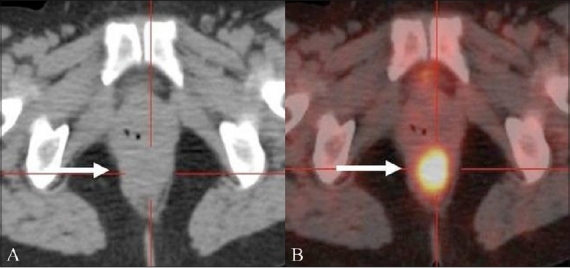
A 60-year-old man treated with surgery for low rectal cancer presented with bleeding per rectum and underwent an FDG PET / CT study. Axial unenhanced CT scan (A) shows ill-defined soft tissue thickening in the perianal region (arrow) without definite evidence of a nodule / mass. Fusion PET / CT image (B) shows an intense focus of hypermetabolism corresponding to the soft tissue thickening, suggesting perianal recurrence
